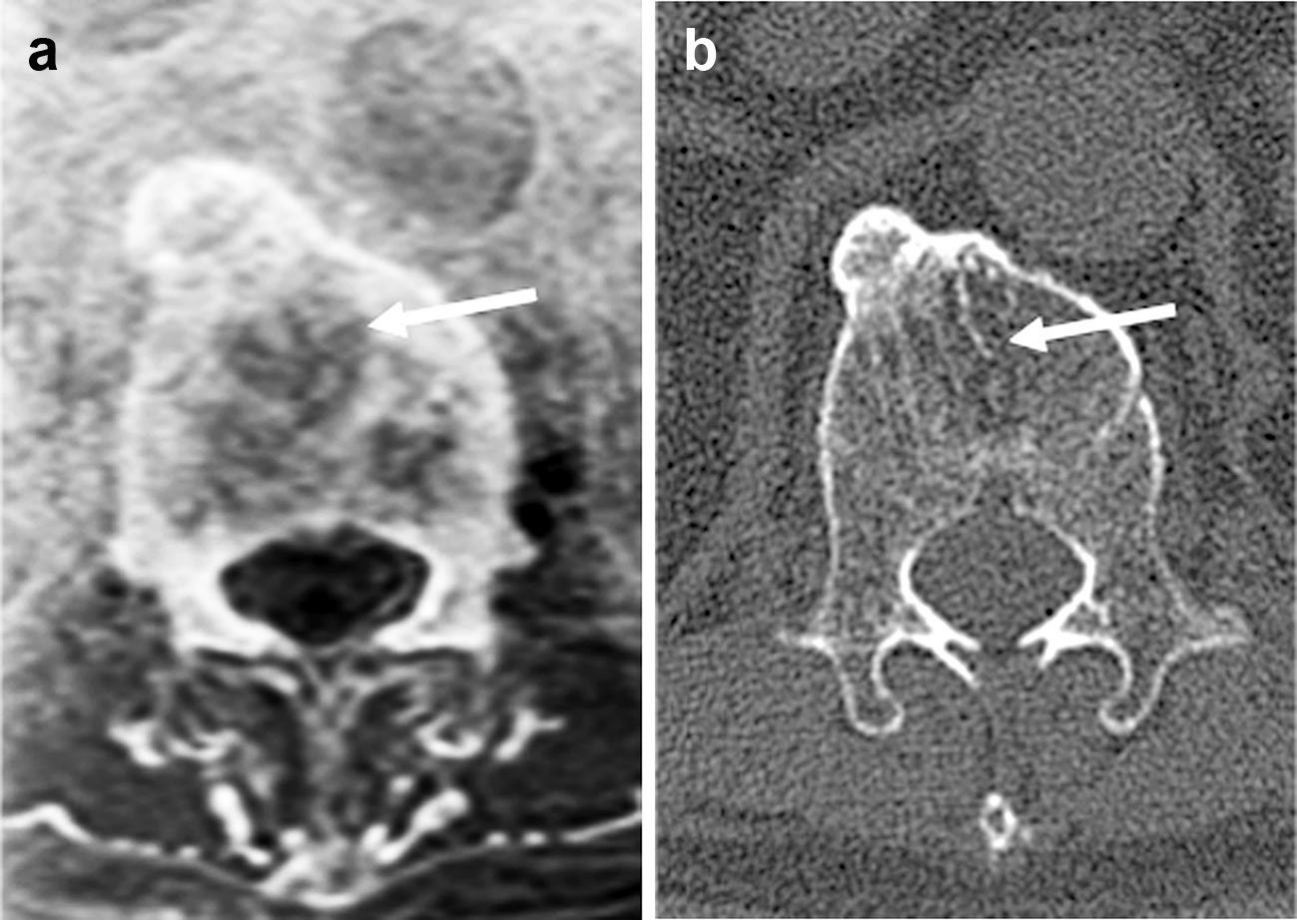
Hemangioma. Axial MR bone image (a) of an 84-year-old male shows a lucent lesion margin (arrow). Thickened internal trabeculae are also well visualized. Axial CT image (b) shows similar findings, but the lesion margin is unclear (arrow)
